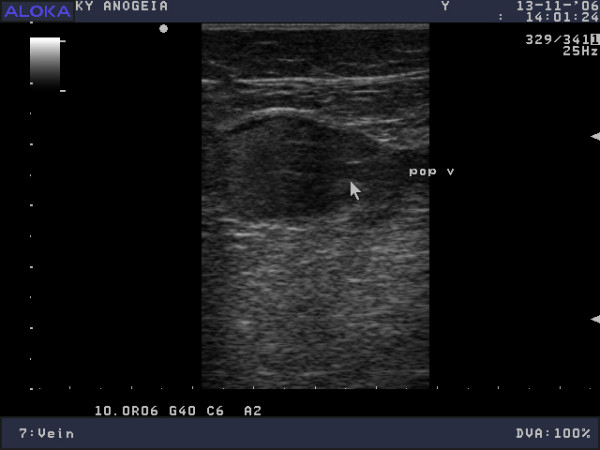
Distal right popliteal vein, B-mode ultrasonogram, transverse axis. The vein lumen could be obliterated using a small amount of extrinsic pressure.
